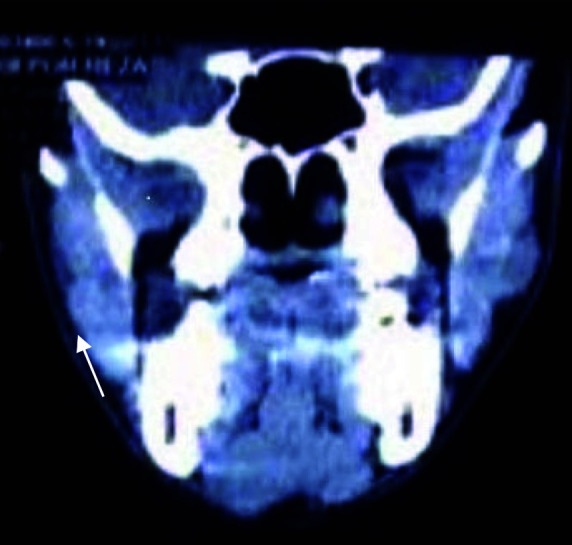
Computed tomography without contrast (coronal view) showing small well-defined hypo dense solid lesion adjacent to the left masseter muscle and in the superficial lobe of left parotid gland
